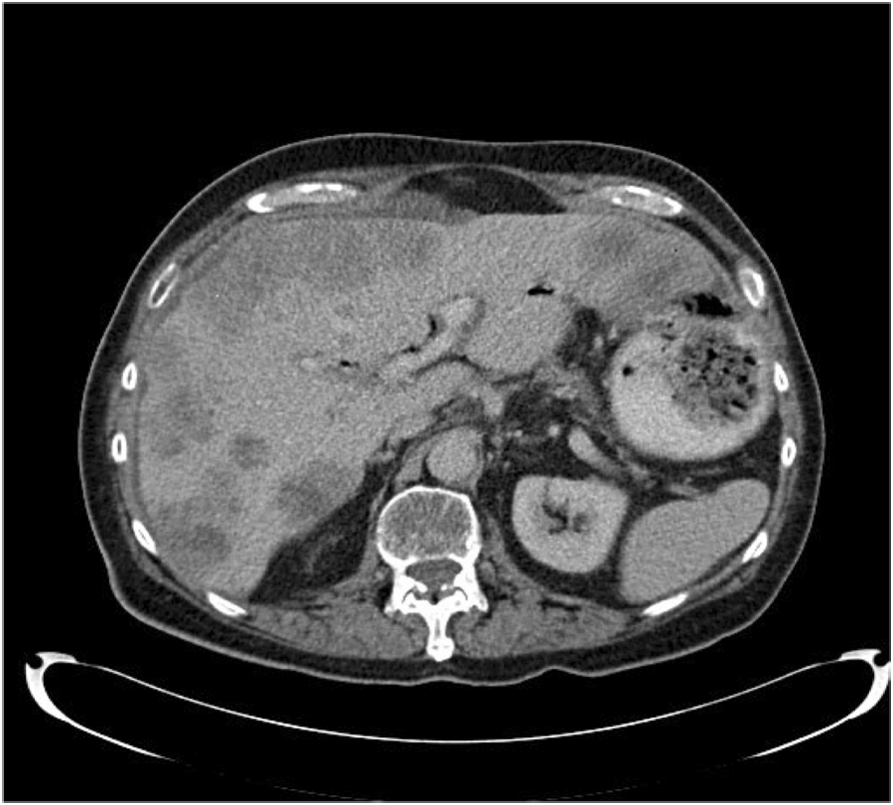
Computed tomography scan showing multiple liver metastases prior to second-line palliative chemotherapy.
